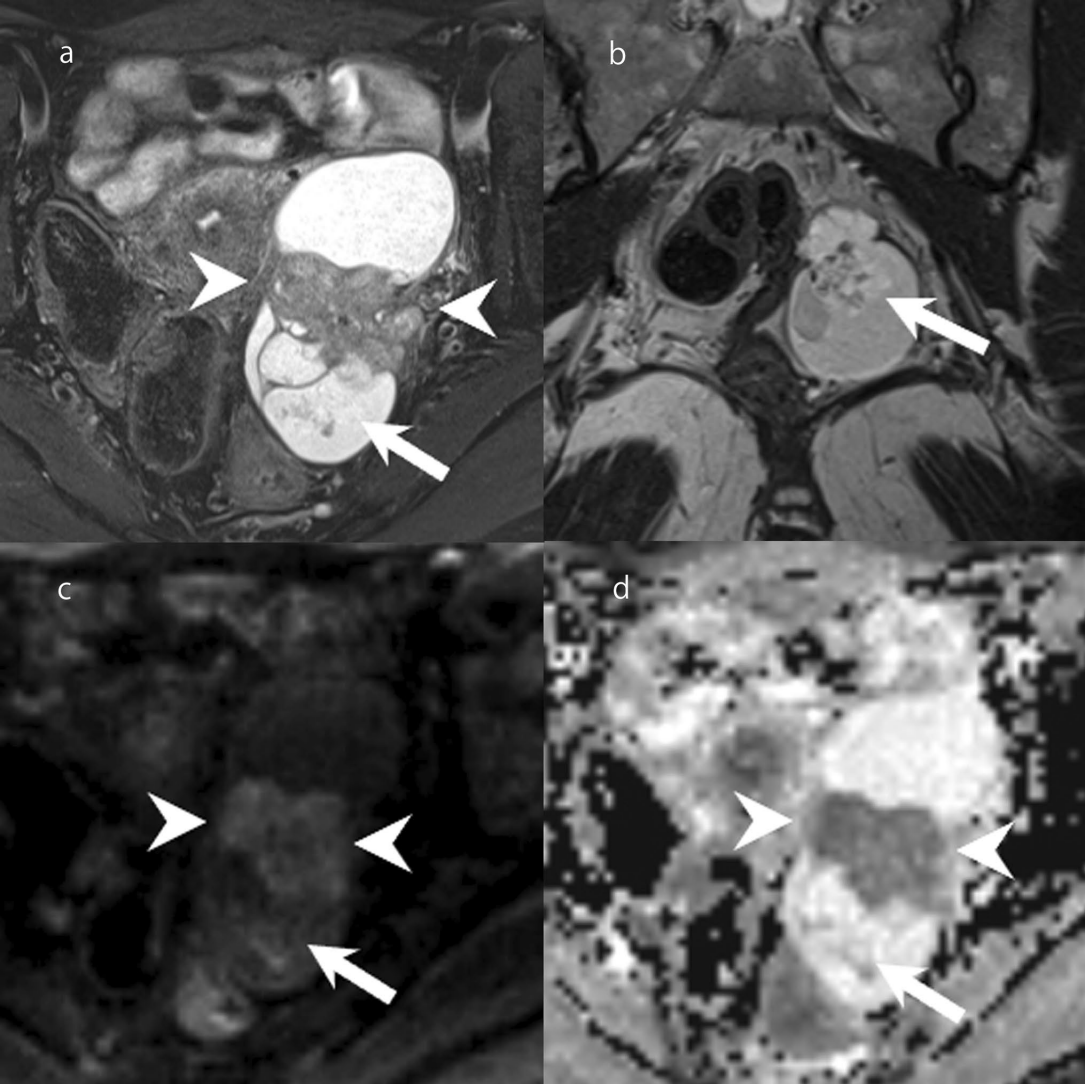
Clear cell carcinoma of a woman in her 40s. a A mass consisting of cysts and solid components was found in the left ovary. The center of the mass has a solid component with moderate hyperintensity on FS-T2WI (arrowhead). There is also a papillary mural nodule in the posterior mass loculus that shows a markedly hyperintense area with a dendritic hypointense area in the center on FS-T2WI (arrow). b A papillary mural nodule is clearly depicted on coronal T2WI (arrow). c On DWI (high *b* value), the solid component in the center of the mass is slightly hyperintense (arrowhead), whereas the papillary mural nodule is hypointense (arrow). d On ADC maps, the solid component in the center of the mass is hypointense (arrowhead), whereas the papillary mural nodule is hyperintense (arrow)
